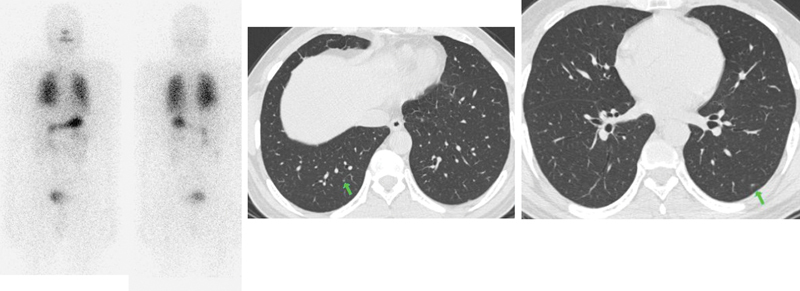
Diffusely increased radioiodine uptake is seen in both lungs with tiny nodules seen on HRCT (
